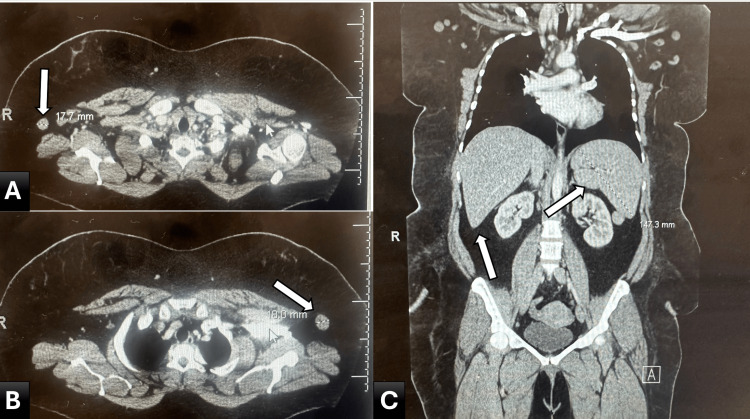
CT demonstrating systemic involvement. (A, B) Axial contrast-enhanced CT images demonstrating bilateral axillary lymphadenopathy (arrows), measuring up to approximately 1.7–1.8 cm. (C) Coronal image demonstrating hepatosplenomegaly (arrows), with splenic enlargement measuring approximately 14.7 cm in craniocaudal dimension. These findings are consistent with systemic involvement in T-lymphoblastic leukemia/lymphoma.
